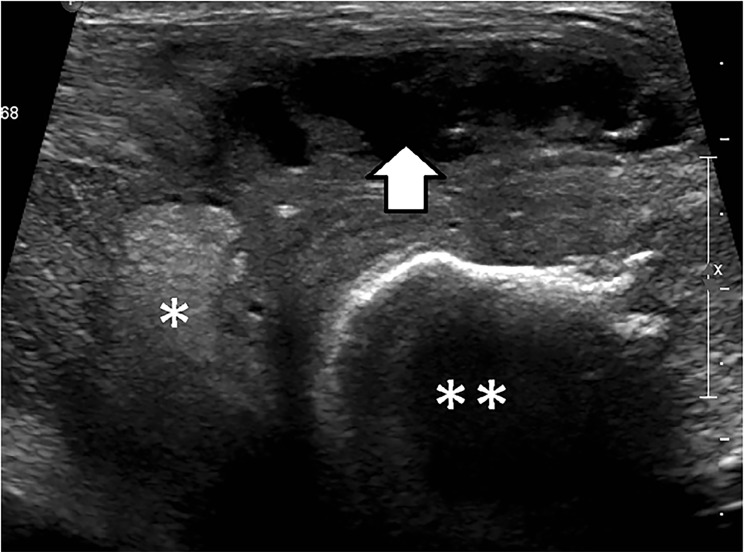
Ultrasound image of the area of pain and erythema between the sternum (*) and the right clavicle (), demonstrating a subcutaneous fluid collection (arrow).
